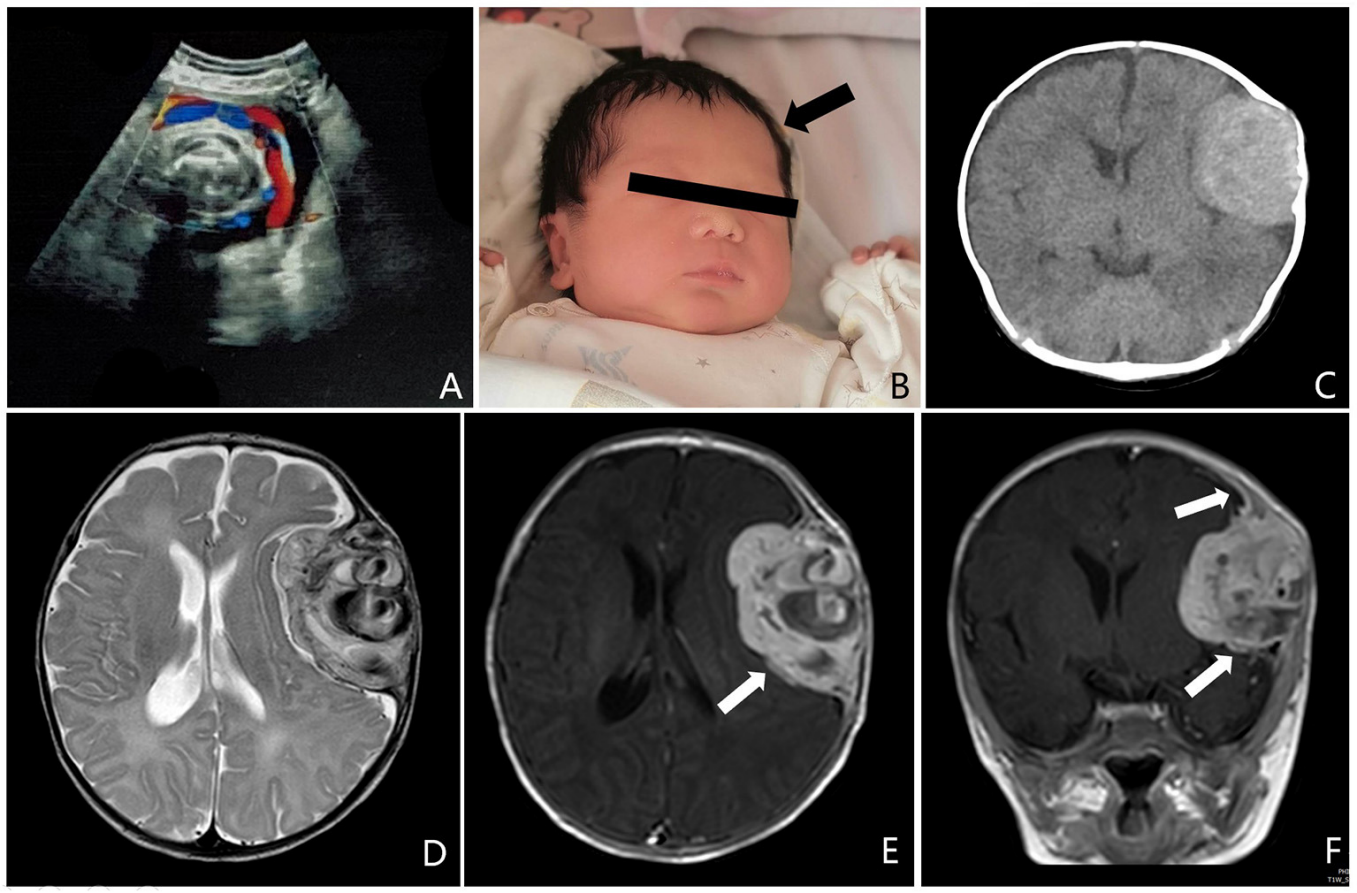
(A) Antenatal ultrasound at 36 weeks of gestation demonstrated a hyperechoic signal was present in the left frontal lobe, with clear borders, and irregular morphology. Low resistance blood flow signal was seen within the abnormal entity. (B) At the age of 15 days, a slight bulge was observed on the left forehead (arrow). (C) Preoperative computed tomography (CT) scans suggested that a massive hematoma was noted under the left frontal skull plate, with a little subdural hemorrhage in the adjacent temporal area. The circumambient brain parenchyma is obviously compressed and the affected skull bulges outward. (D–F) Preoperative brain magnetic resonance (MR) examination found a huge epidural mass under the left frontal skull, with a heterogeneous internal signal, and multiple patchy low signals. The adjacent meninges enhanced and thickened on T1 MRI after enhancement (arrow).
